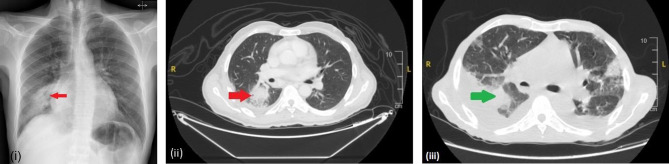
Image (i) A chest radiograph upon arrival of the first admission, demonstrates a right lower zone infiltration with a small cavitary lesion. Image (ii) CT chest of the first admission, demonstrate a 2.7 × 3 cm heterogenous enhancing soft tissue lesion compressing the right lower lobe segmental bronchus (red arrows). Image (iii) CT Chest of the second admission, show the evolving right lower lobe mass reaching 10.3 × 10.5 cm making more compression on the right lower segmental main bronchus (green arrows) with the presence of air pockets as demonstrated as shown in images
